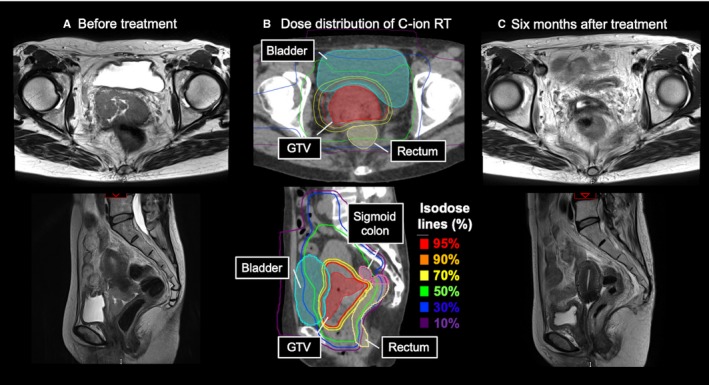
The representative case treated with carbon‐ion radiotherapy. Axial and sagittal MRI T2‐weighted images at before treatment are shown in (A). Dose distributions on CT images are shown in (B). Axial and sagittal MRI T2‐weighted images at 6 months after treatment are shown in (C). Abbreviations: C‐ion RT, carbon‐ion radiotherapy; CT, computed tomography; GTV, gross tumor volume; MRI, magnetic resonance imaging
